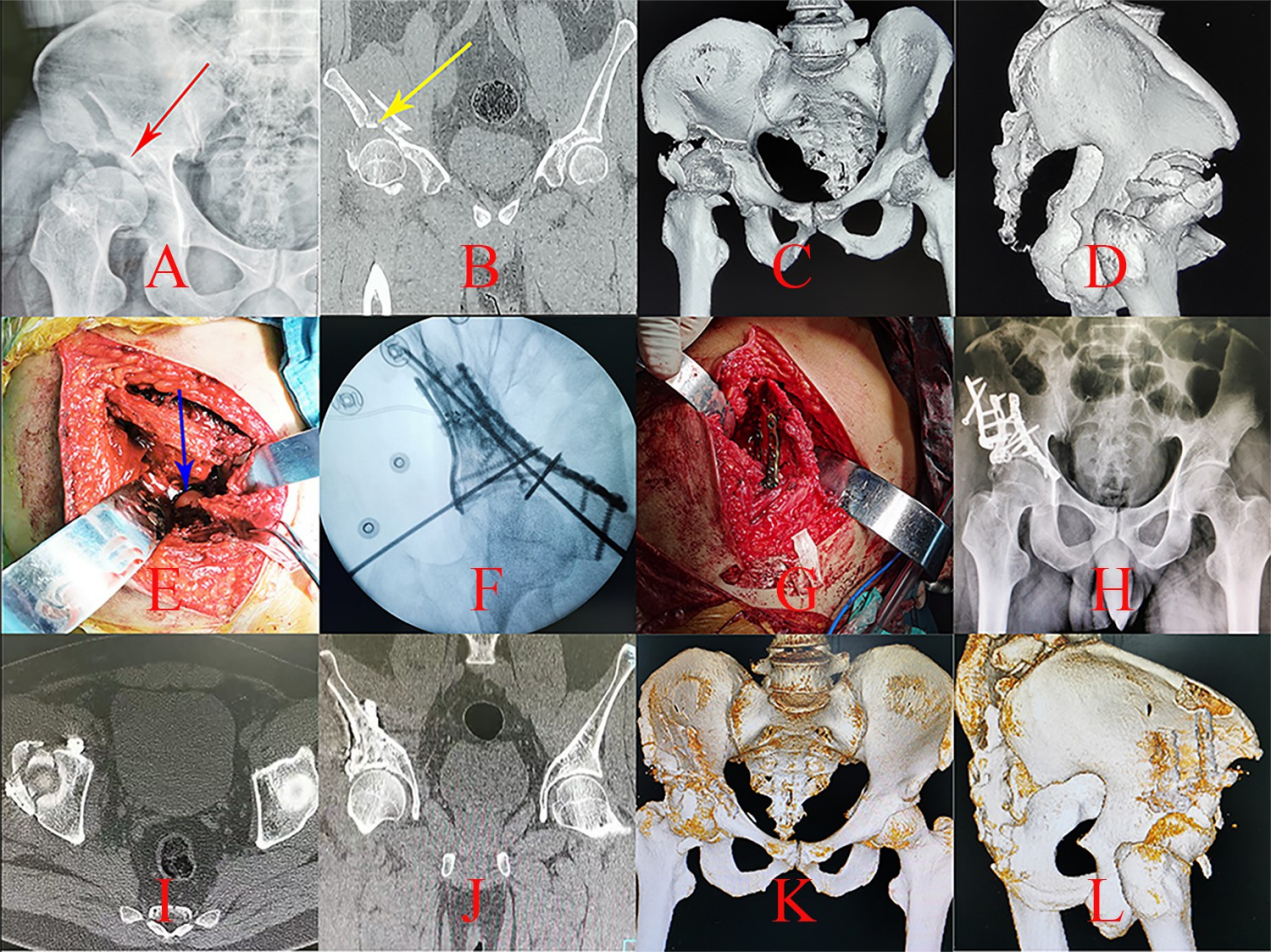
A 3.3 injury patient with articular surface compression who received iliac crest osteotomy through the iliofemoral technique was presented. A, gull sign (red arrow) in preoperative AP view; B, articular surface compression (yellow arrow) in preoperative CT scan; C-D, detachment of roof column and wall on preoperative 3D reconstruction; E, femoral head (blue arrow) could be observed after iliac crest osteotomy, which could serve as a landmark for the reduction of gull fragment; F, reduction of articular surface compression during intraoperative projection; G, intraoperative view of fixation; H, imperfect reduction quality on postoperative AP view; I-J, the reconstructed acetabulum presented a larger diameter than the corresponding femoral heads and the contralateral acetabulum on postoperative CT scans; K-L, fracture fixation on postoperative 3D reconstruction.
